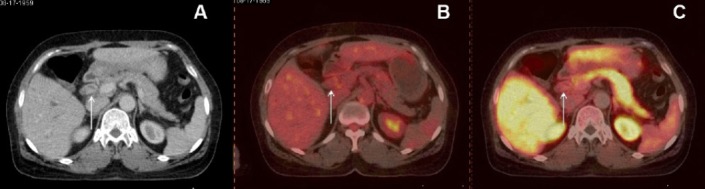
A 52-year-old woman with well differentiated extrahepatic CCA. A) Transaxial contrast-enhanced CT shows an intraluminal, irregular, enhancing soft tissue lesion. B) Transaxial ^11^C-choline and C) transaxial ^18^F-FDG PET/CT images show no significant uptake in the lesion with T/B ratios of 0.6 and 0.78, respectively
